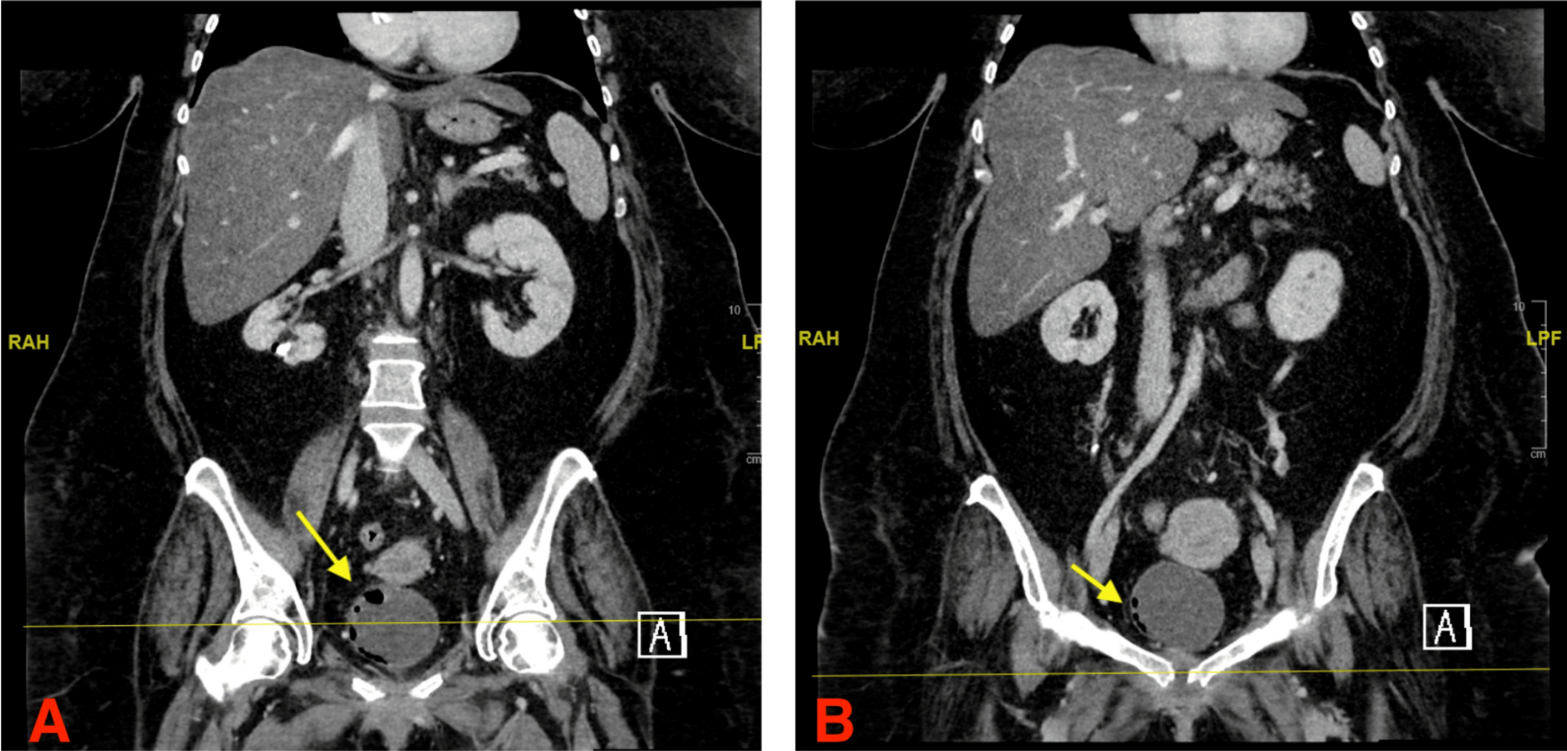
CT scan images (A and B) show pneumatosis of the urinary bladder wall (depicted by yellow arrows) The findings are compatible with emphysematous cystitis CT: computed tomography
